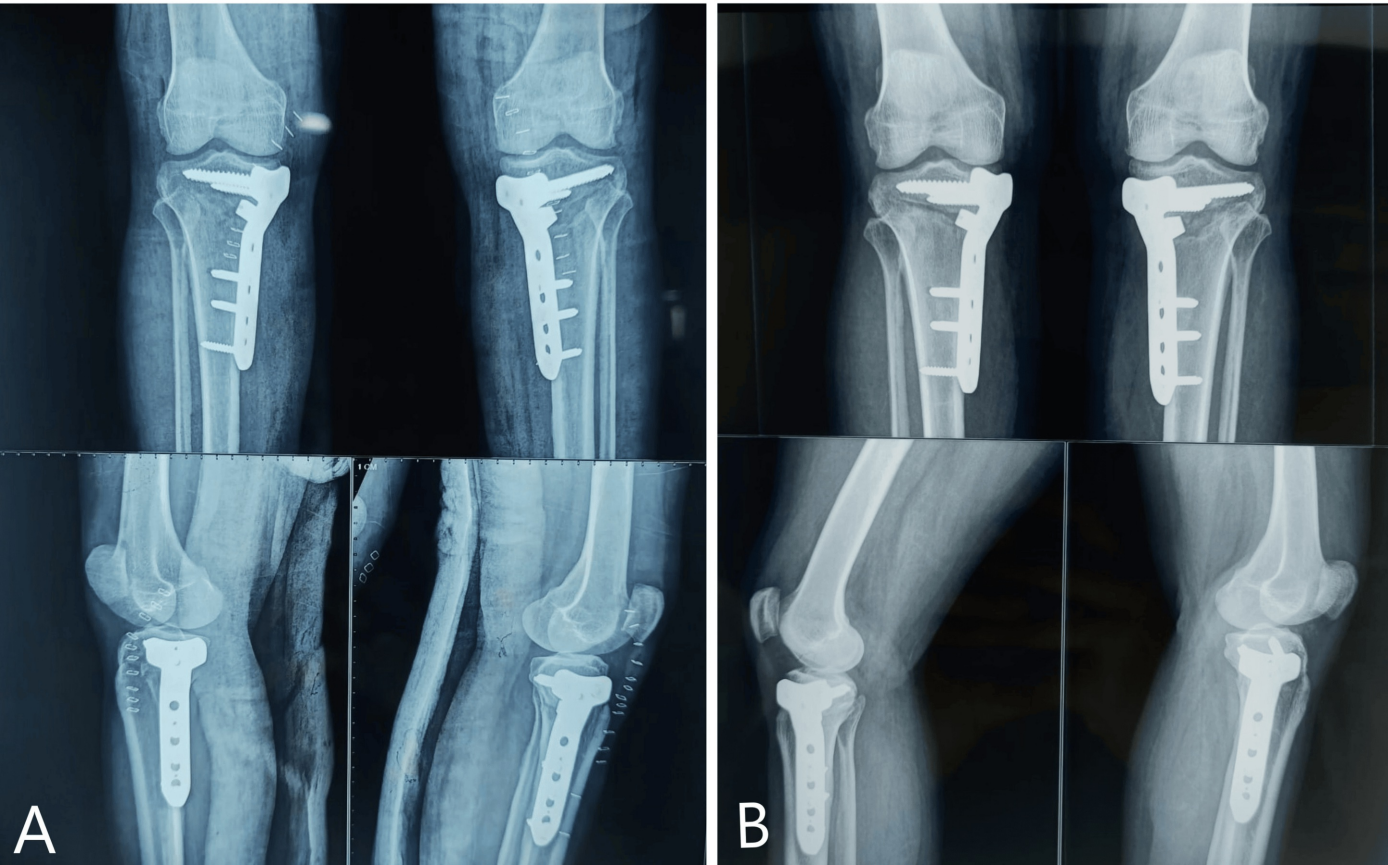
A) Immediate post-operative X-ray showing Implant in good position and the deformity was corrected to acceptable range. B) Three months follow-up X-ray showing remodeling at the osteotomy site.
